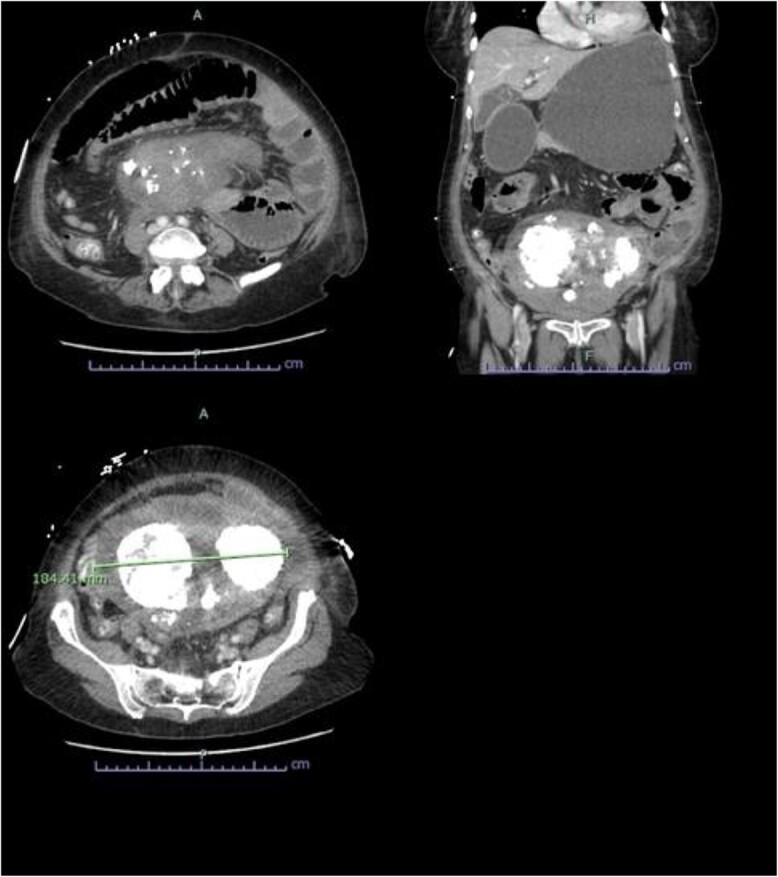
Abdominopelvic CT findings of a large multifibroid uterus with amorphous central calcification associated with a small bowel obstruction.
